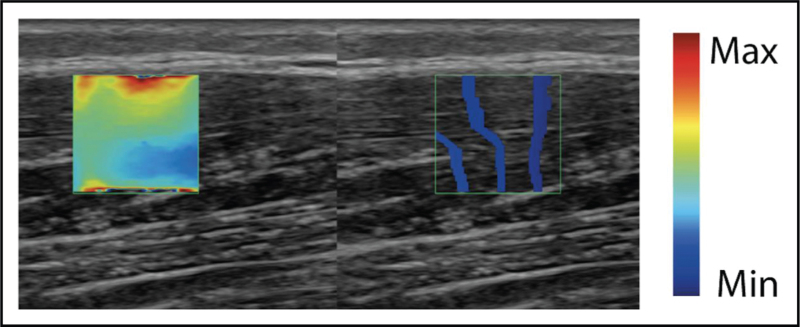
Shear wave elastography. Example of shear wave elastography ultrasound images. A high intensity pulse produces shear waves which propagate laterally. Tracking of laterally propaged shear waves with low intensity pulses allows to measure shear wave velocity, which is a measure of tissue elasticity.
